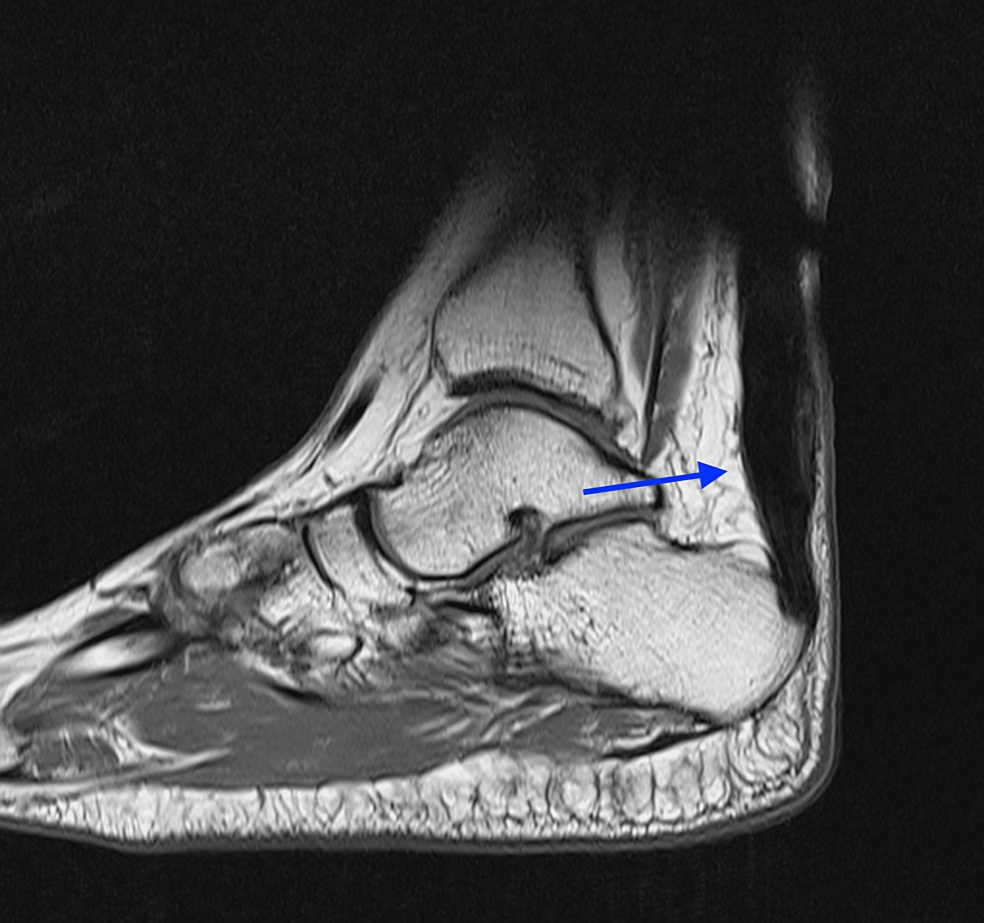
MRI of the right foot: A T1 weighted image depicting complete healing of the repair (blue arrow)
